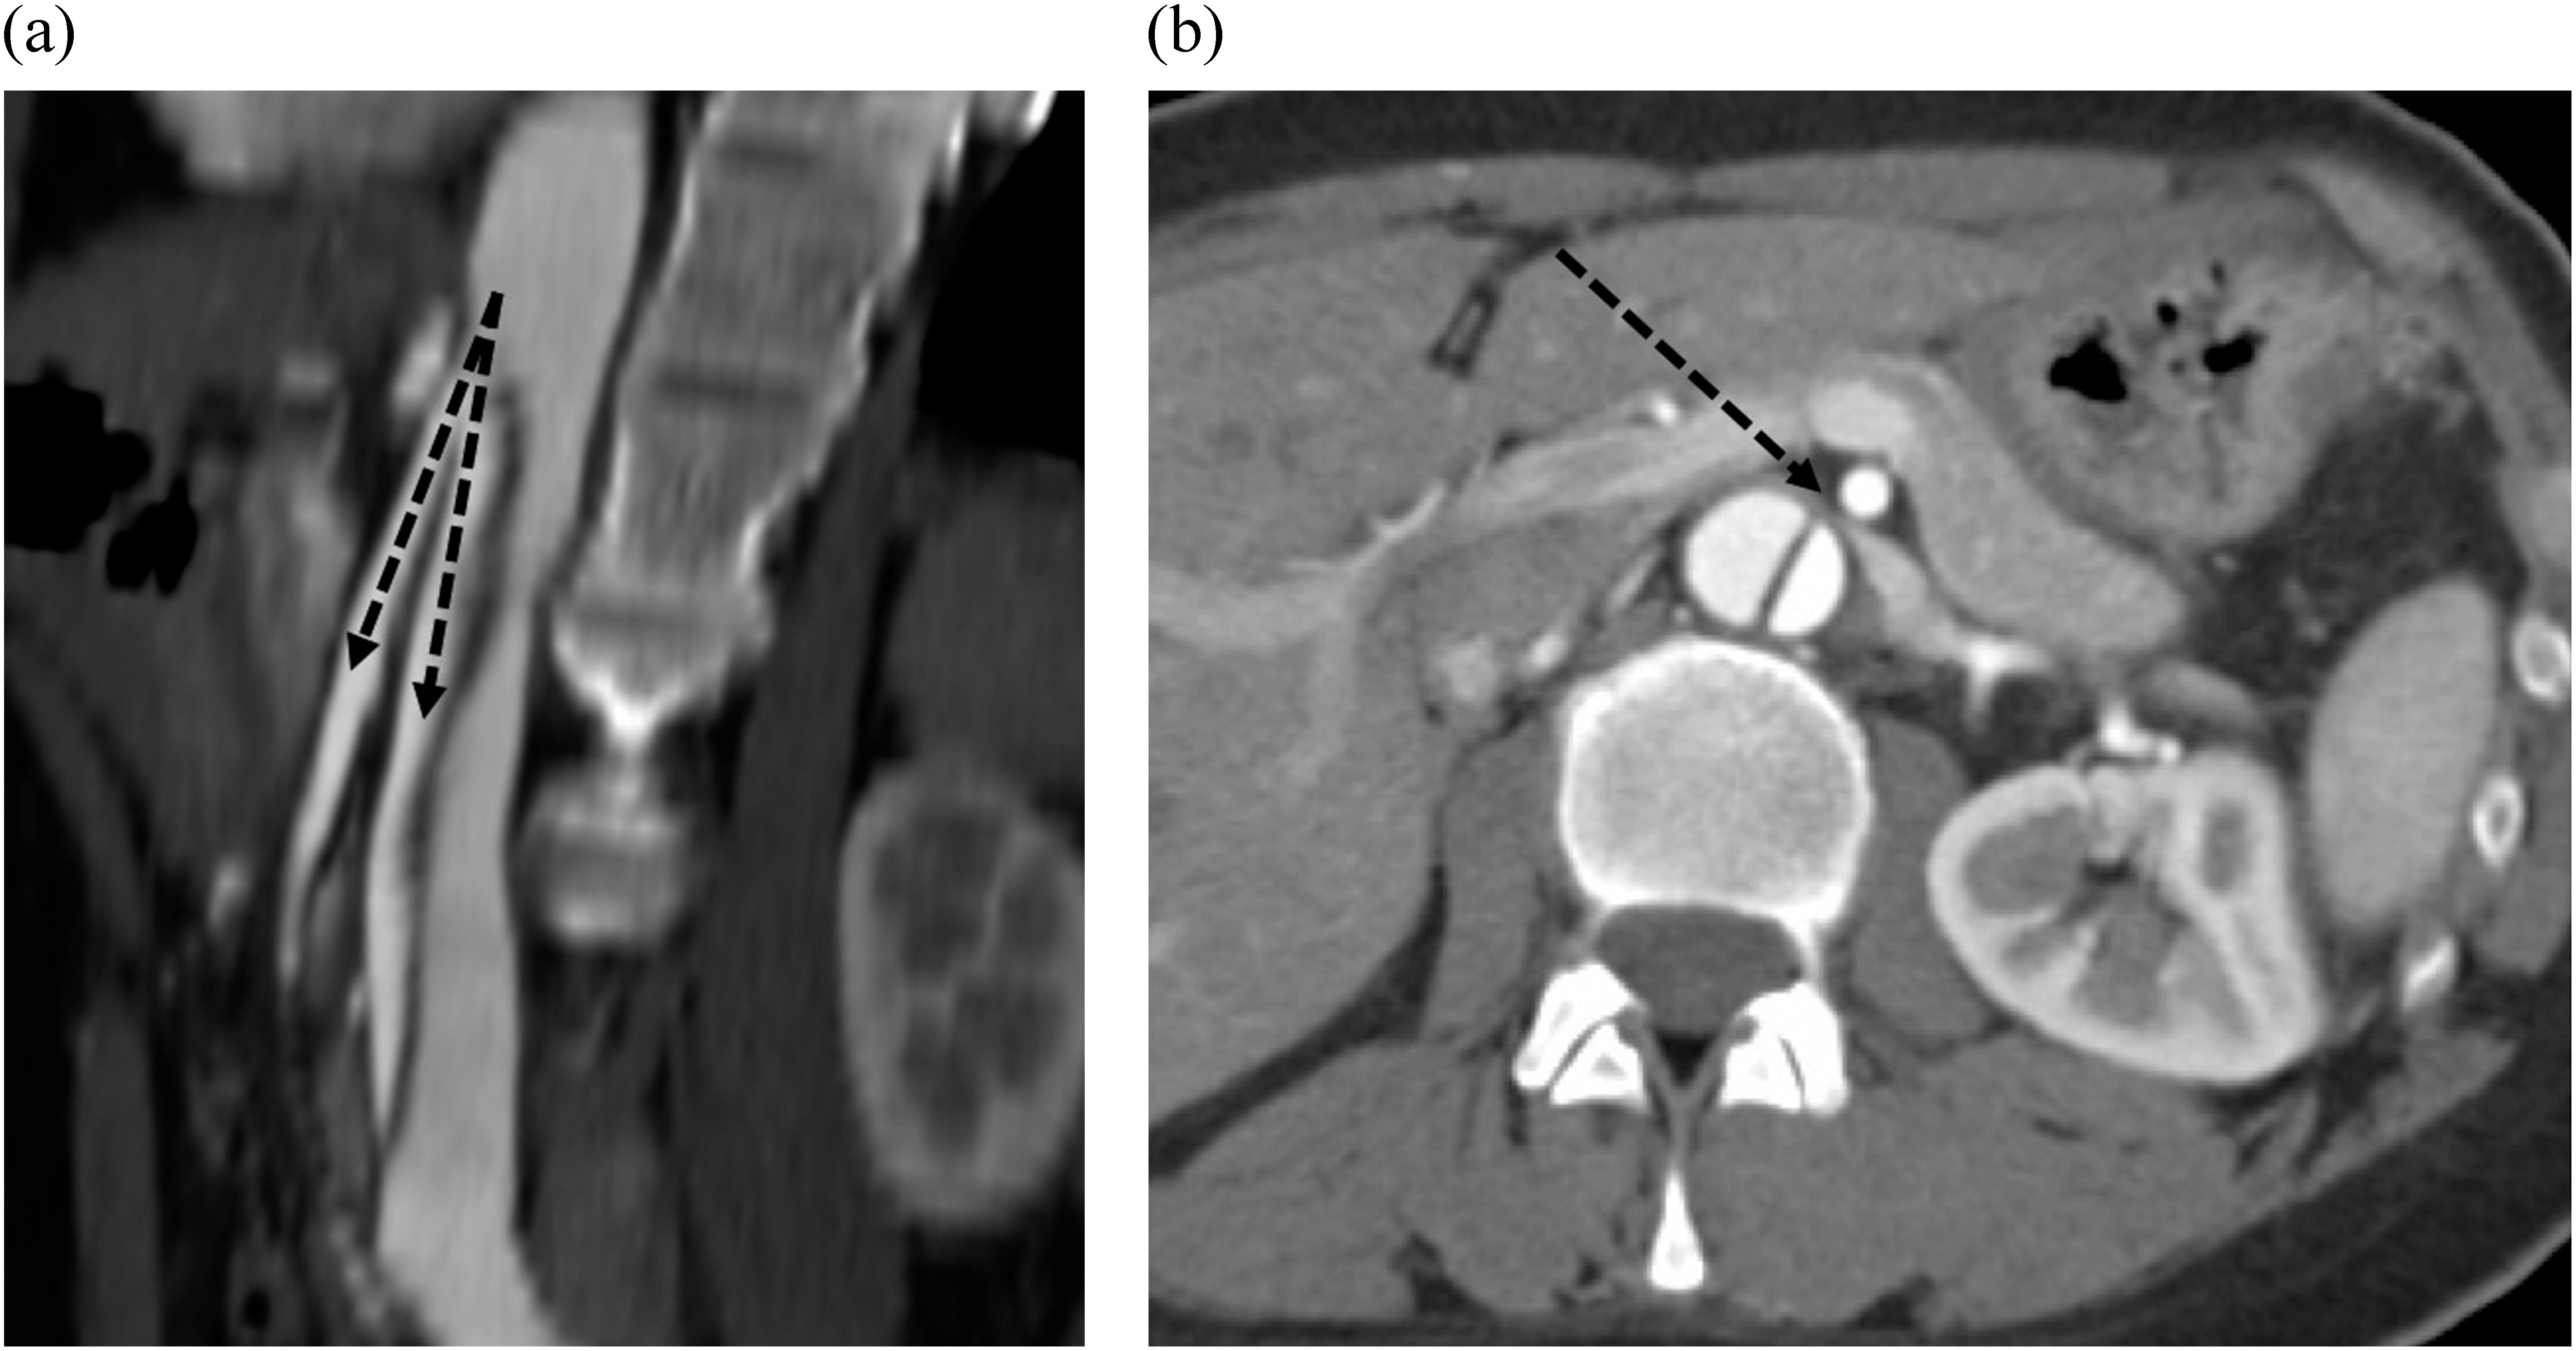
Fig. 2 (a) A preoperative computed tomographic scan revealing the sharp aortomesenteric angle (dotted arrows). (b) A preoperative computed tomographic scan revealing the short aortomesenteric distance (dotted arrow).
